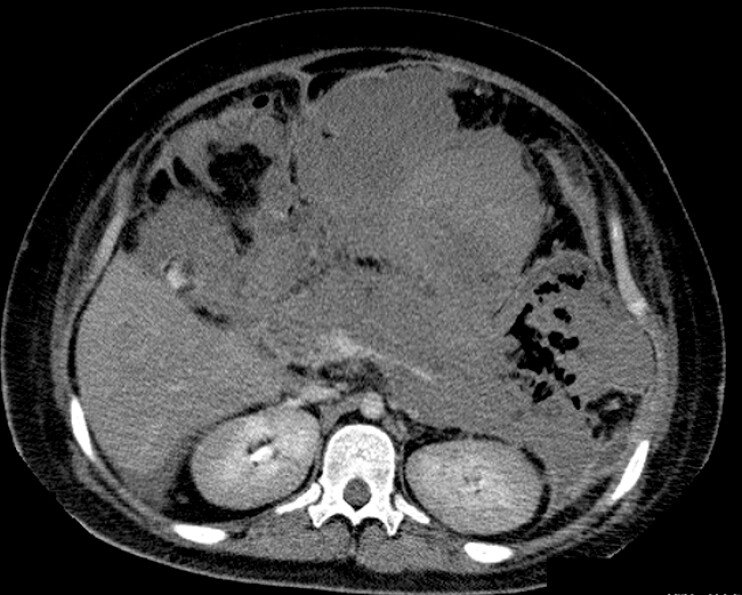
Computed tomography on day 42 of pancreatitis showed extensive walled-off necrosis. The lesion extended caudally into the pelvic cavity prior to intervention.
